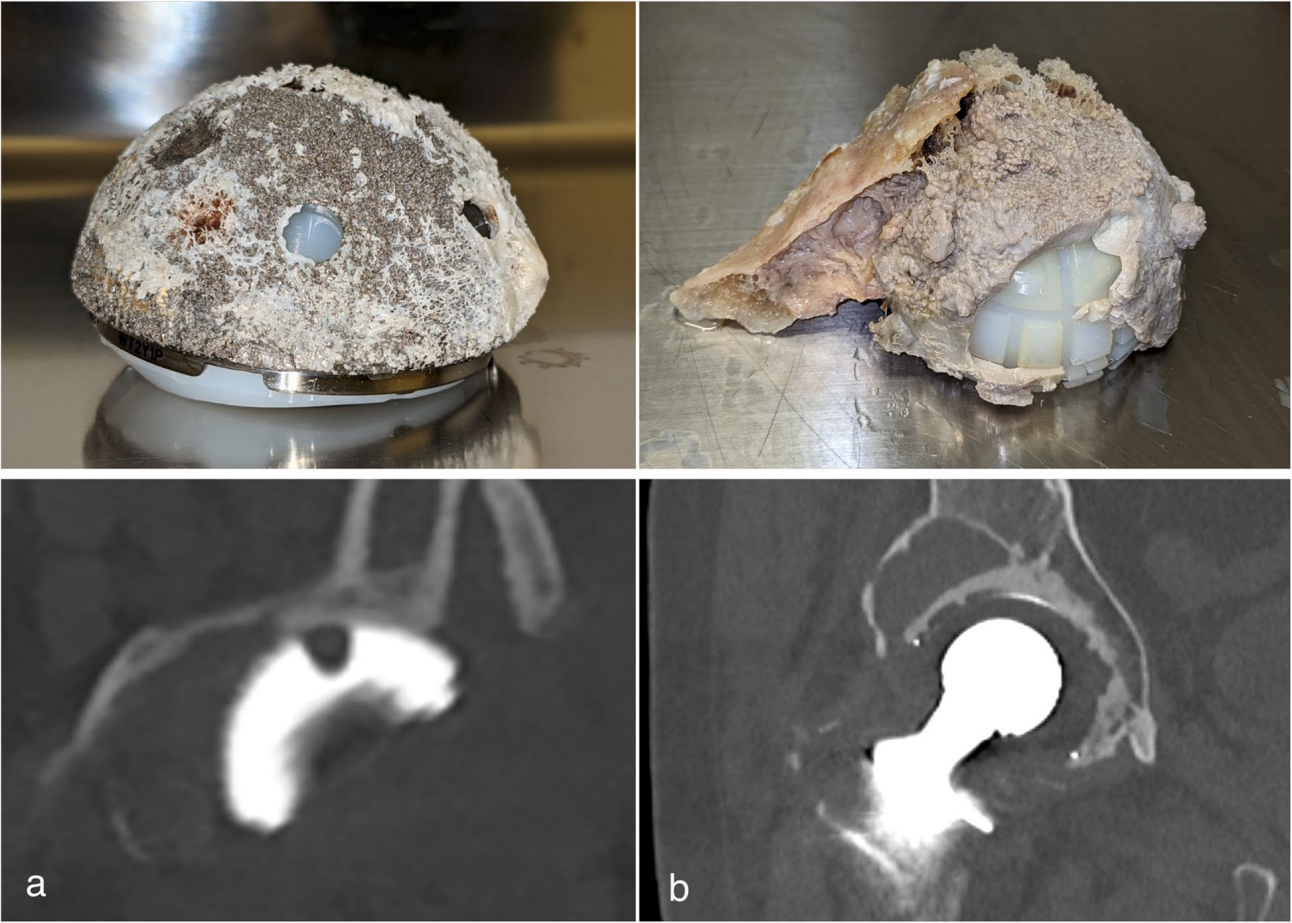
Retrieved uncemented (a) and cemented (b) acetabular cup implant and clinical computed tomography images acquired before revision surgery (lower row)
